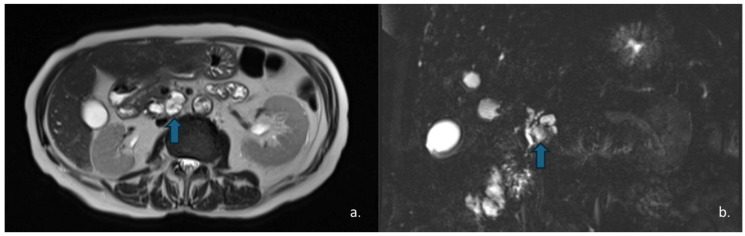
MRI T2-w HASTE (a) and MR cholangiographic (b) sequences show a hyperintense lesion at the level of the pancreatic uncinate process (arrow). The cholangiographic sequence demonstrates communication with the main pancreatic duct (duct of Wirsung), consistent with a type II intraductal papillary mucinous neoplasm (IPMN).
